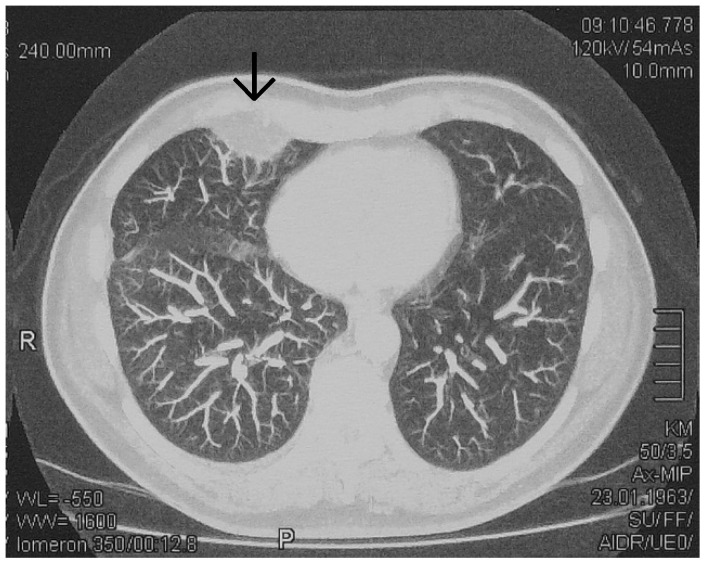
Computed tomography pulmonary angiogram (CTPA) showing an area of subpleural PI (arrow) in the right lower lobe.
